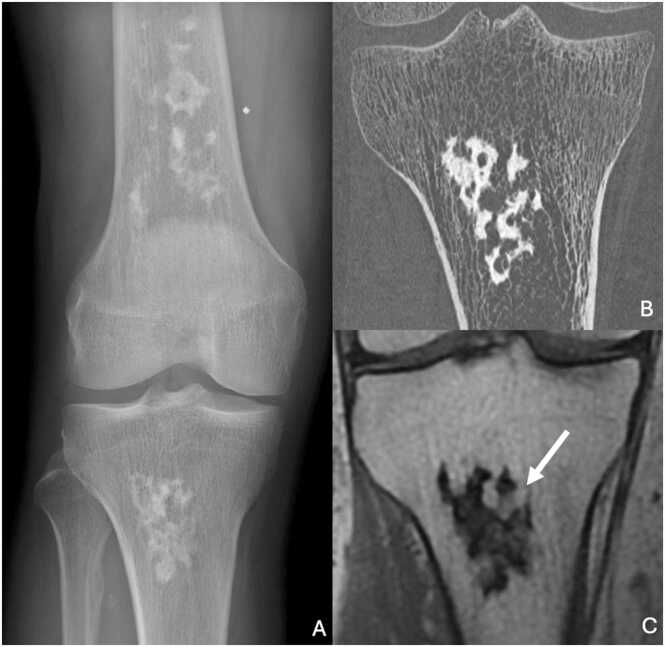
Bone infarcts of right femur and tibia in a 41-year-old man. A. Face radiography showing serpiginous sclerotic femoral and tibial lesions, producing a “smoke volute” appearance. B. UHR CT image in the coronal plane higlighting the serpiginous sclerotic ostemedullar lesion of proximal tibia. C. T1-weighted Dixon (in phase) MR image in the coronal plane showing a marked hyposignal of the lesion corresponding to sclerotic portions and some fatty areas with signal similar to adjacent normal osteomedullar bone (white arrow).
